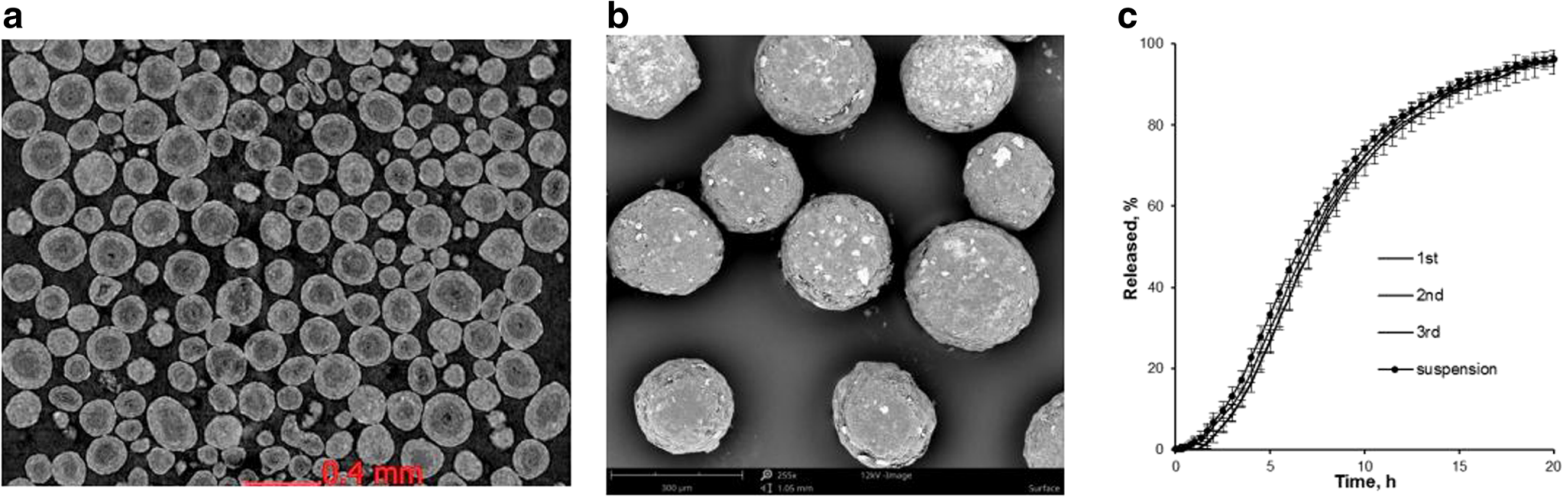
Structure of metoprolol succinate-loaded and Eudragit® NM 30 D-coated MPs: virtual cross-sections using computed tomography of MPs (A); SEM picture of entire MPs (B); and drug release profiles of three batches (1^st^, 2^nd^ and 3^rd^) of metoprolol succinate MPs coated with F10 and after 30 min storage post reconstitution into liquid suspension (C).
